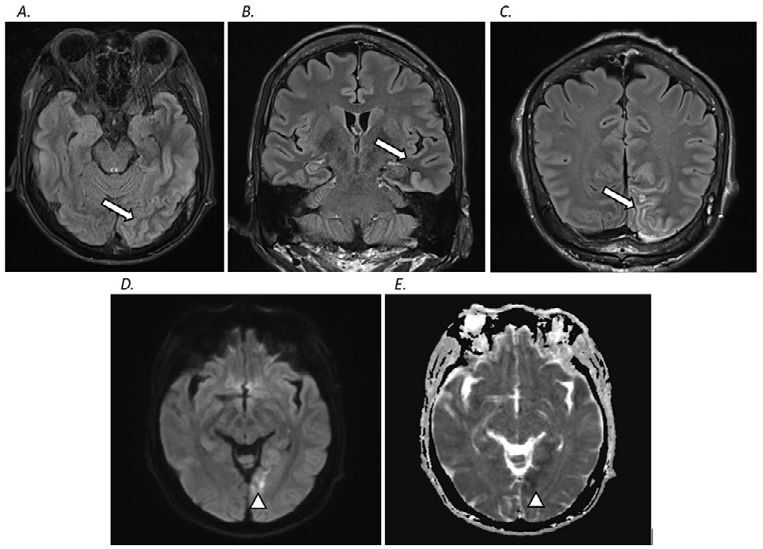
The 3-tesla brain MRI of the patient showed mild cortical hyperintense and subcortical hypointense T2/FLAIR lesions expanding from the left mesial temporal, inferior temporal, and occipital areas (arrows) [FLAIR; A – axial view, B and C – coronal view]. Restricted diffusion was presented at the left mesial temporal area with involvement of the left hippocampal gyrus (arrowheads). [D – DWI axial view, E – ADC axial view].
